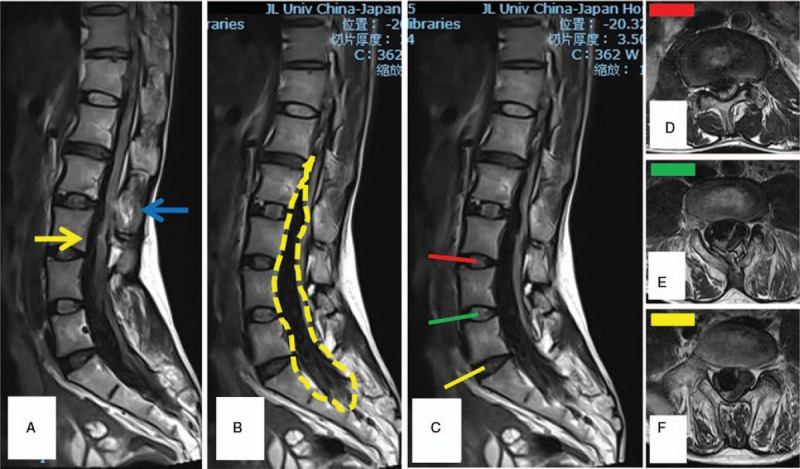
(A) The sagittal T2-weighted image (T2WI) magnetice resonance imaging (MRI) showed the conus medullaris was at L3/4 intervertebral level (yellow arrow). The anesthesia puncture site was at L2/3 intervertebral level with T2WI hyperintense signal on the interspinous ligament (blue arrow). (B) On T2WI MRI, massive spindle-like intraspinal T2WI hypointense signal masses spread from T12 to S2 ventrally (yellow dotted line). (C–F) The sagittal T2WI MRI showed the massive spindle-like intradural mass compress the spinal cord and cauda equina badly on each segments.
